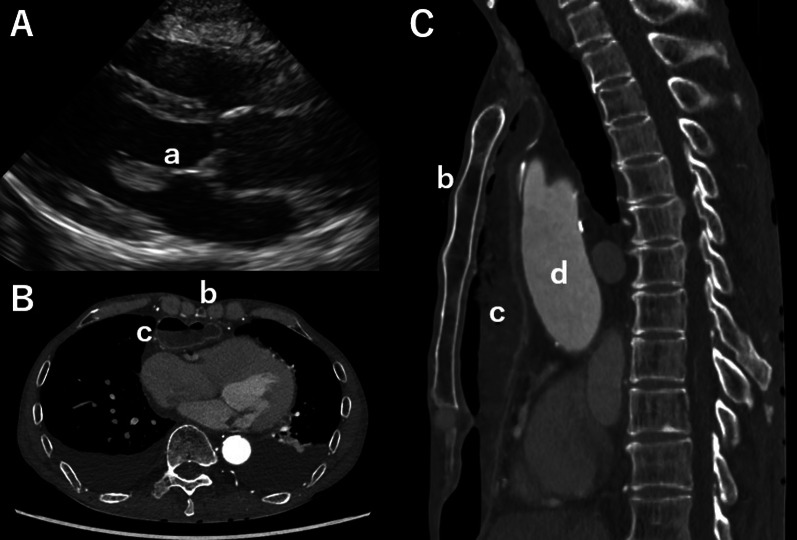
Preoperative transthoracic echocardiography and contrast-enhanced computed tomography findings. A Parasternal long-axis view showing a vegetation attached to the anterior leaflet of the mitral valve (a). B An axial image of the relationship of the sternum (b) and gastric tube (c). C A sagittal image of the conduit and its relationship with the ascending aorta (d)
